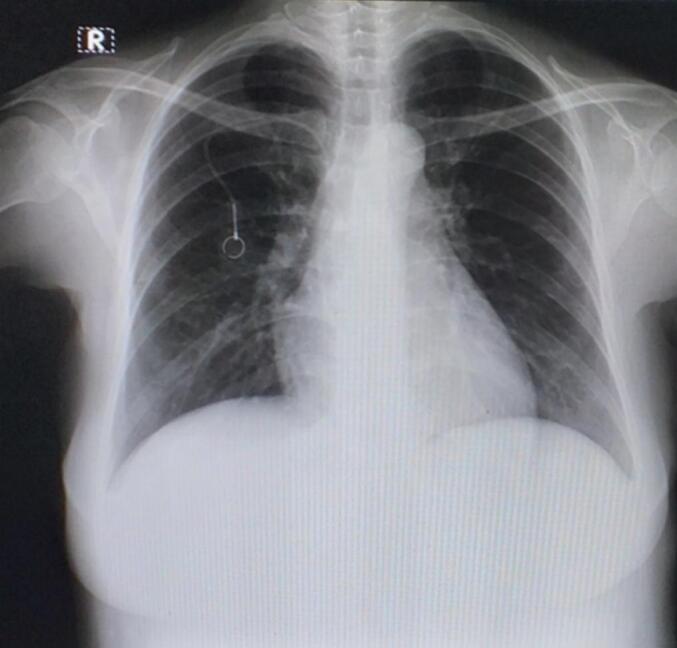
Thoracic X-ray 2-months post-TIVAP insertion.
